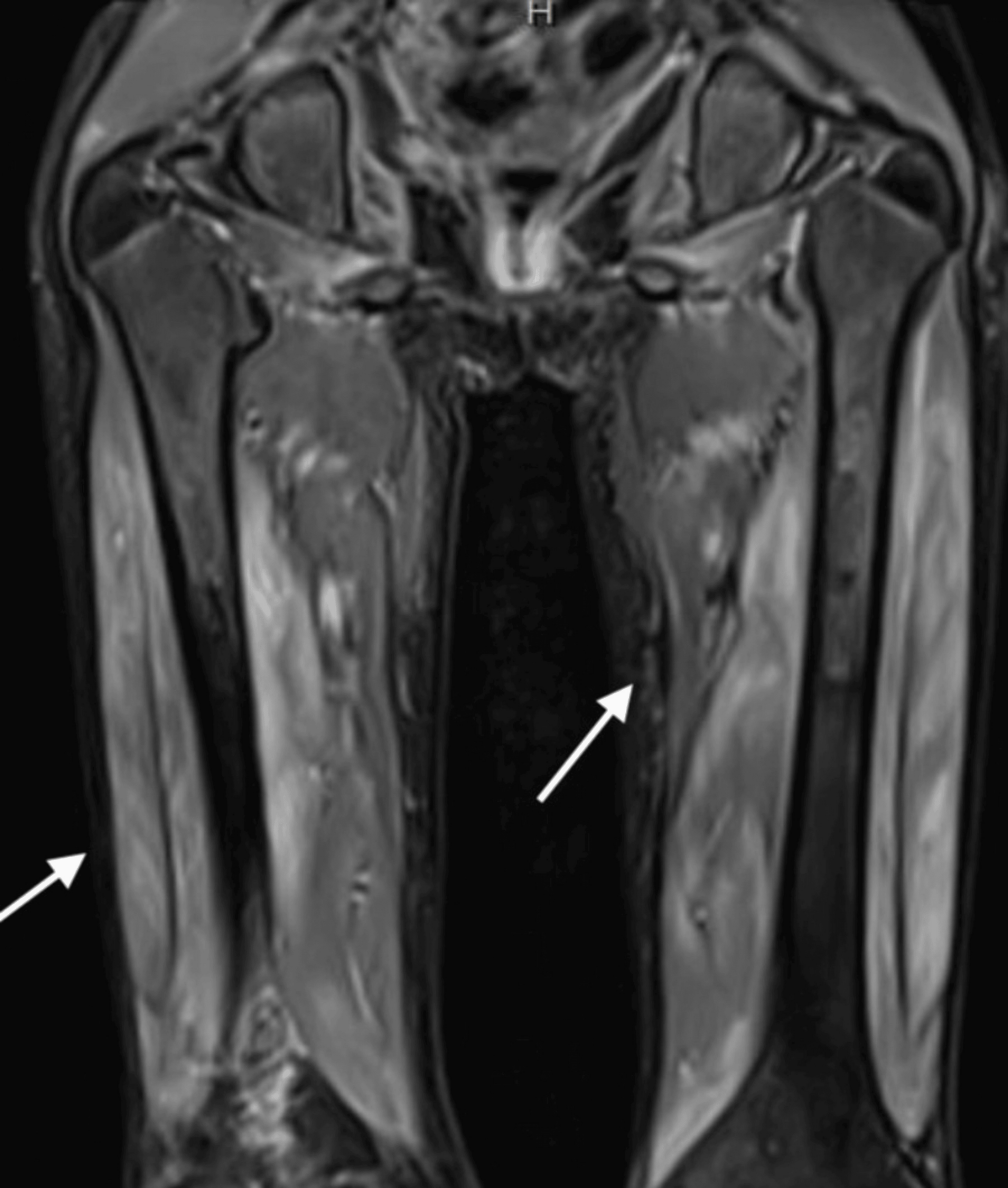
MRI of the thigh showing enhancement of the subcutaneous tissue after contrast administration
